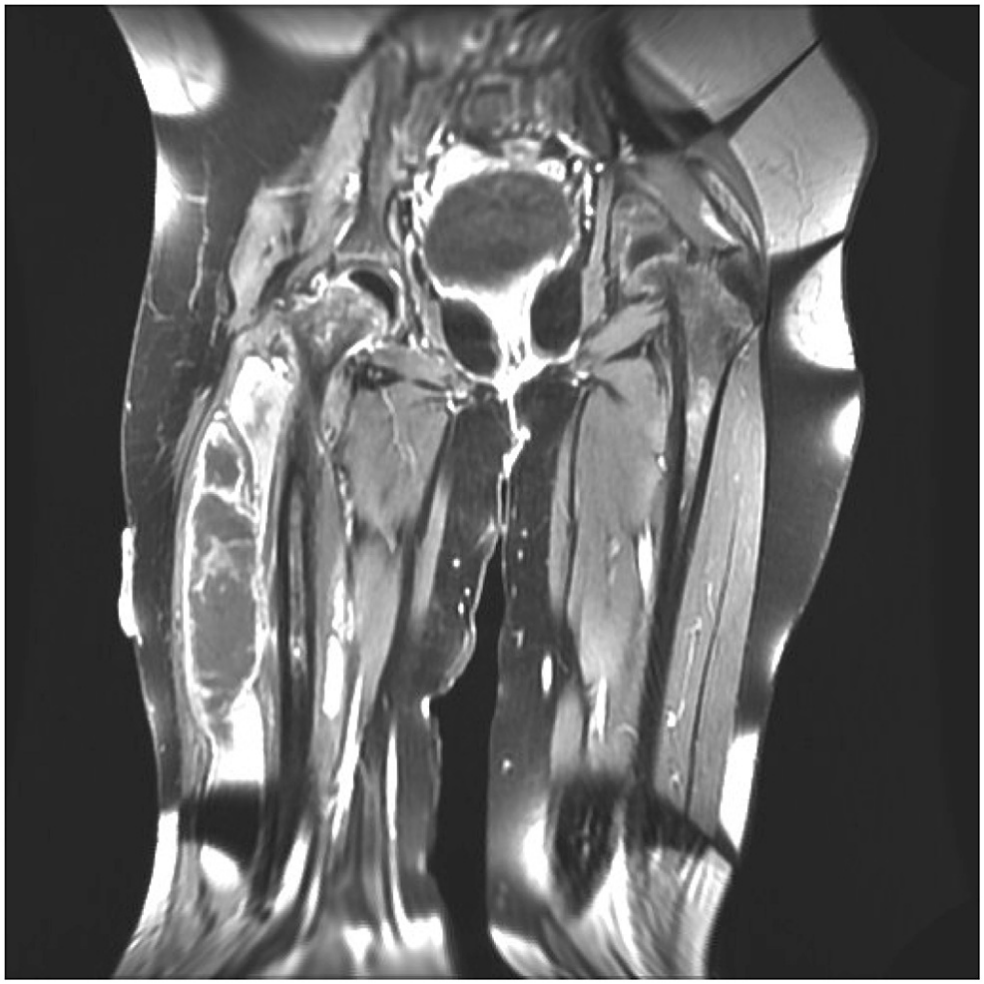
Enhanced magnetic resonance image of the right thigh and hip (sagittal view). The image shows severe subcutaneous and muscle edema with diffuse enhancement of the anterolateral muscle compartments and secondary fasciitis with no signs of septic hip arthritis or osteomyelitis.
